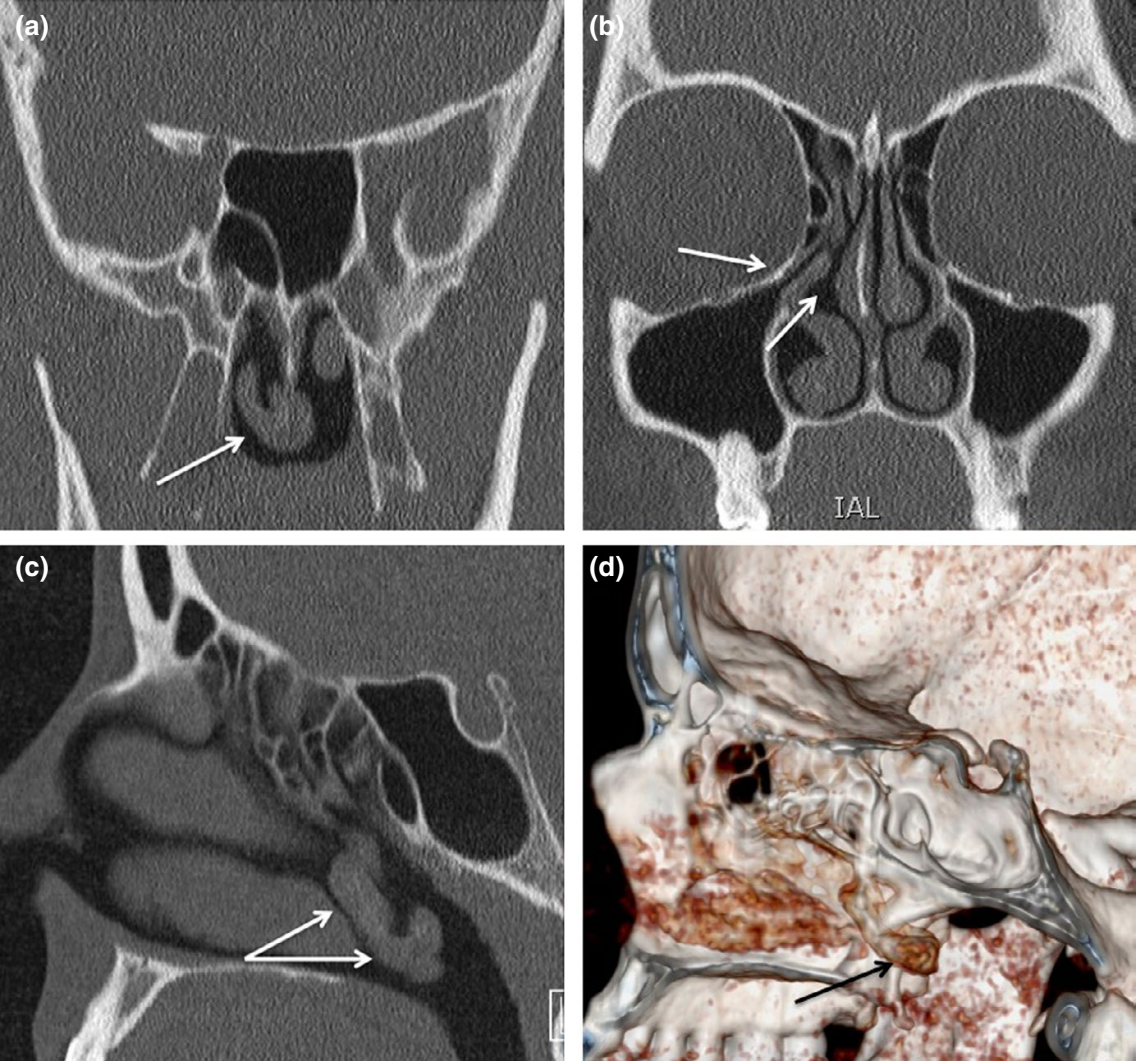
CT Scan reporting the anatomy of the posterior middle turbinate. The coronal (A, B), sagittal C, and 3D reconstruction D, showed the posterior development of the right middle turbinate. The anatomy of uncinate process and right middle meatus was normal B.
